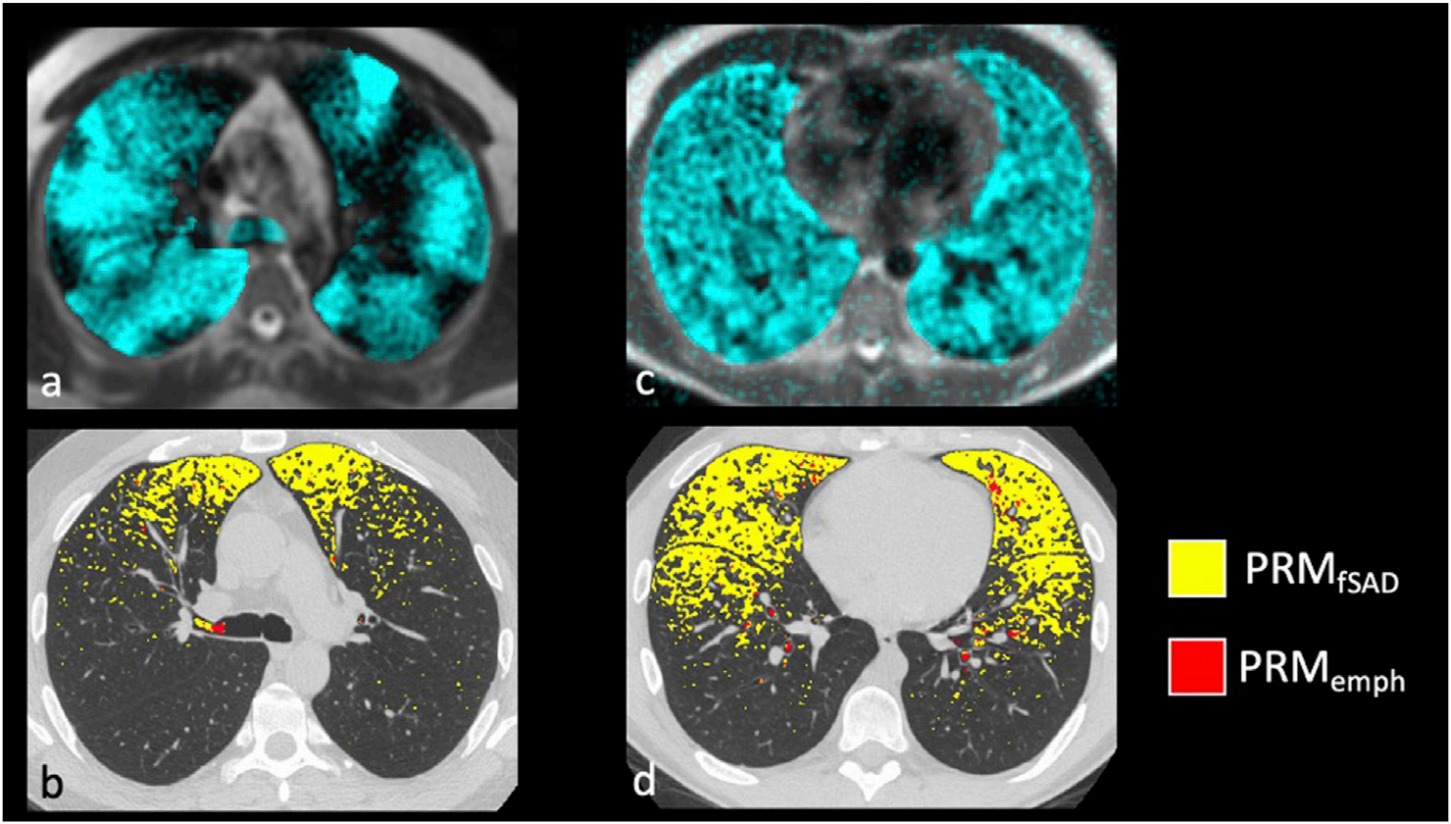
Examples of severe asthmatics where PRM and HP gas MRI are discordant. The most common discordant pattern is (A,B) HP 3He MRI ventilation > fSAD with whole lung VDP of 18.4% and PRMfSAD of 5.8%, and the least common is in (C,D) HP 3He MRI ventilation << fSAD with a whole lung VDP of 6.3% and PRMfSAD of 23.7%.
